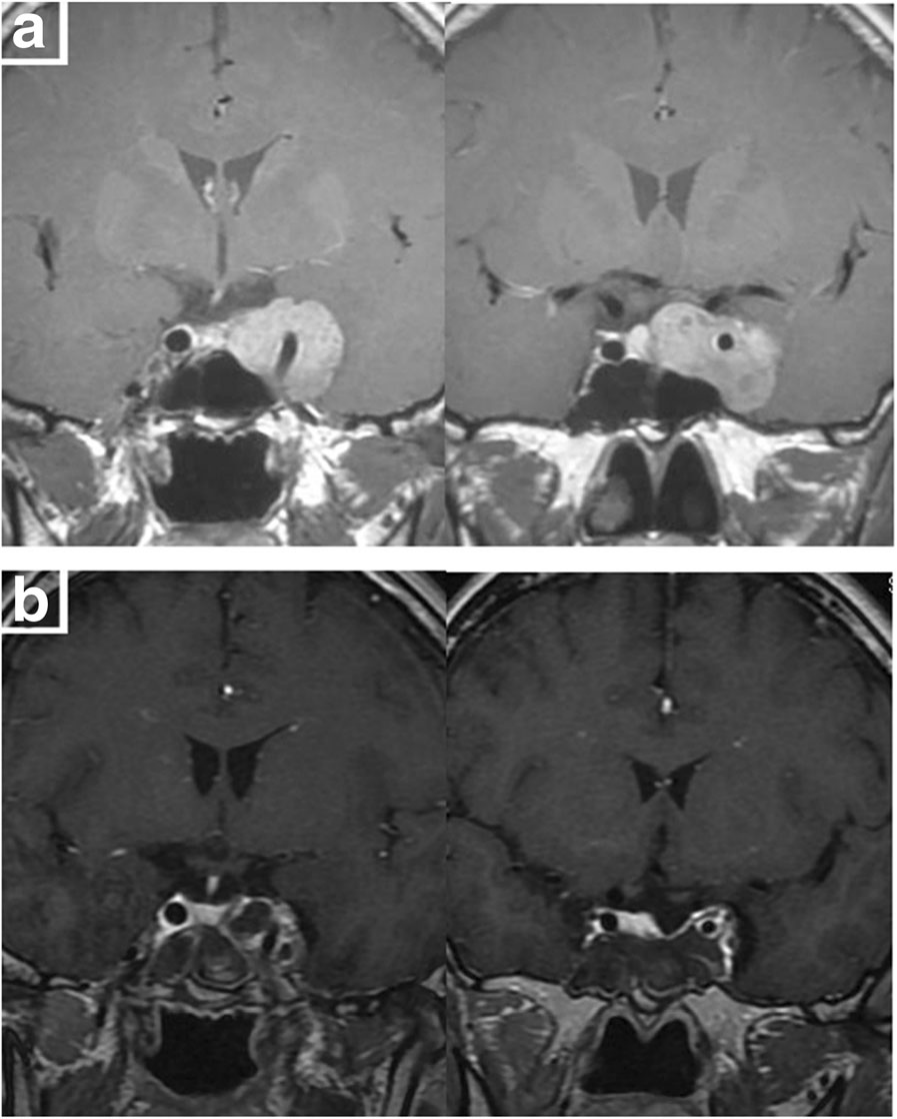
a Preoperative MRI shows left cavernous sinus invasion, encasing left internal carotid artery. b Postoperative MRI shows the tumor invading into cavernous sinus was mostly excised
